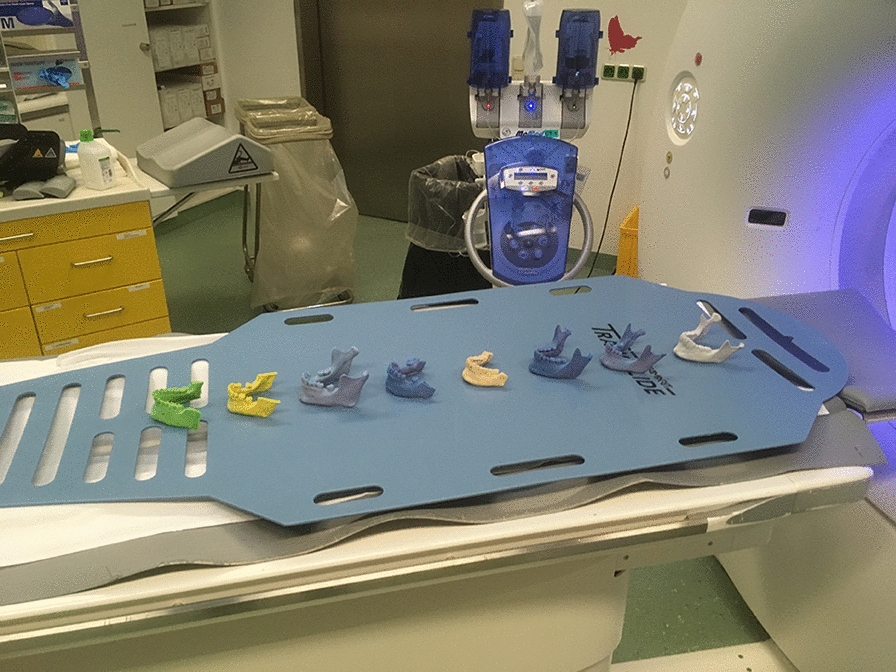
The figure shows customized 3D-printed models in the CT scanner
